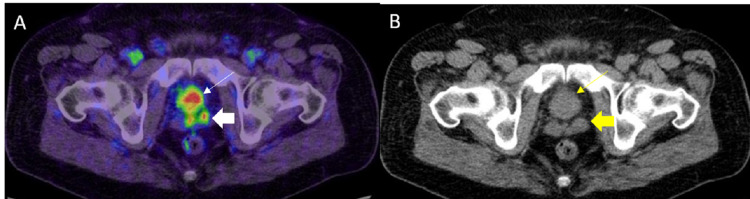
Seminal vesicle invasion A: 68Ga PSMA PET/CT axial image shows uptake in the primary prostate cancer (white thin arrow). Note the invasion of the seminal vesicle (white thick arrow). B: Correlate low-dose axial CT shows the prominent prostate (yellow thin arrow) and seminal vesicle (yellow thick arrow). It is difficult to assess the malignant involvement of the seminal vesicle on this nondiagnostic CT.
